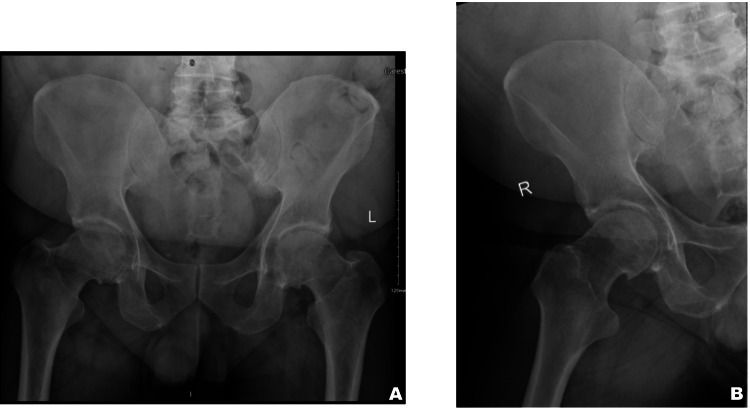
Pelvic and right hip radiographs showing no evidence of acute fracture.
